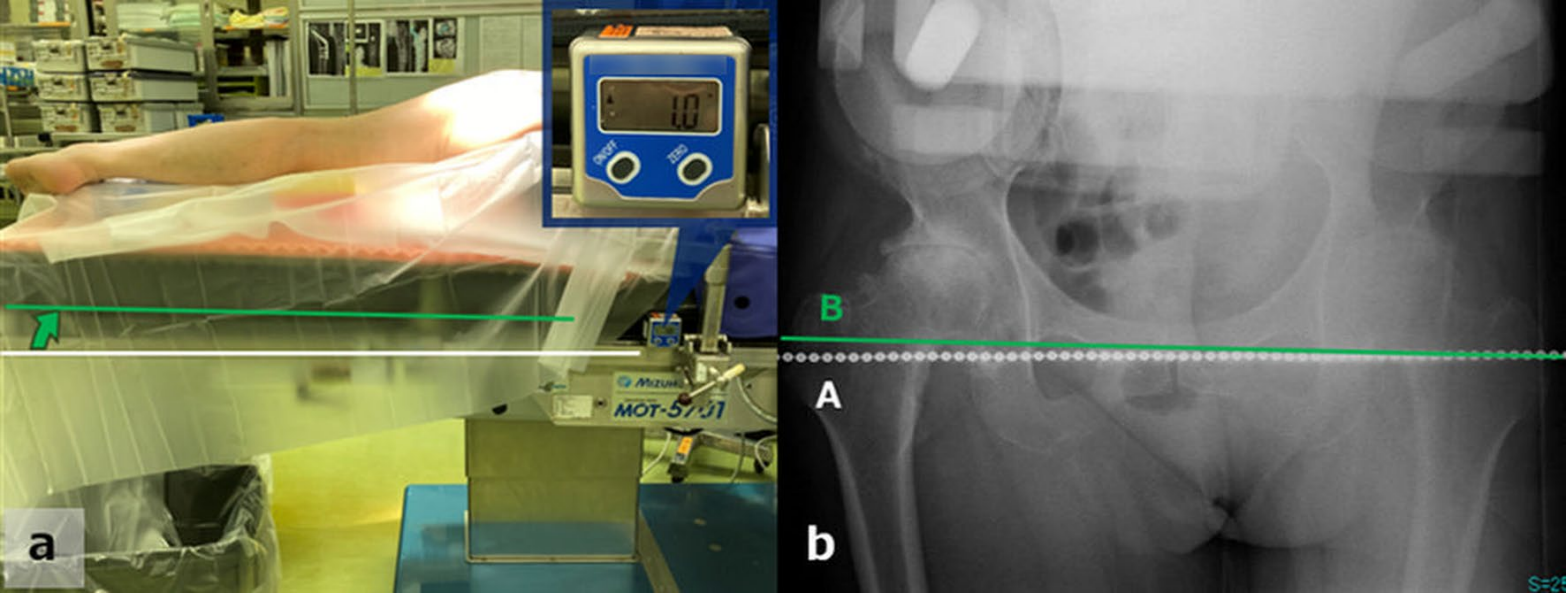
(a) The operating table was tilted 1° cranially, in this case, to adjust the pelvis to the functional pelvic plane. (b) This tilting angle (pelvic tilt in the coronal plane) is measured as an angle formed by a perpendicular line to the floor (a hanging chain, line A) and a teardrop line (line B).THA with laser guide technique using a radiographic cup alignment guide (November 2012–March 2016): Since November 2012, a radiographic angle cup alignment guide (RI 40° and RA 20°) has been introduced. When a laser beam and this alignment guide are parallel and a level gauge indicates 0° in both the coronal and axial planes, the cup is supposed to be in RI 40° and RA 20° (Fig. 5).
